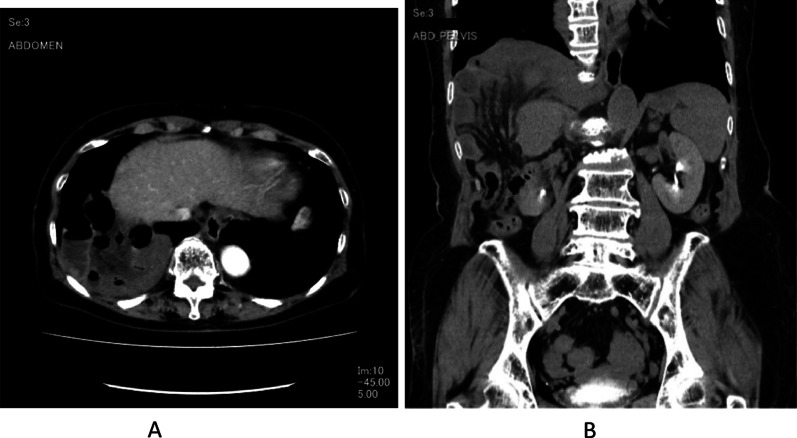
A Contrast-enhanced computed tomography (CT). Contrast-enhanced CT showing herniation of the small intestine into the thoracic cavity. B Coronal CT image. Coronal CT images showing loops of the small intestine in the thoracic cavity
